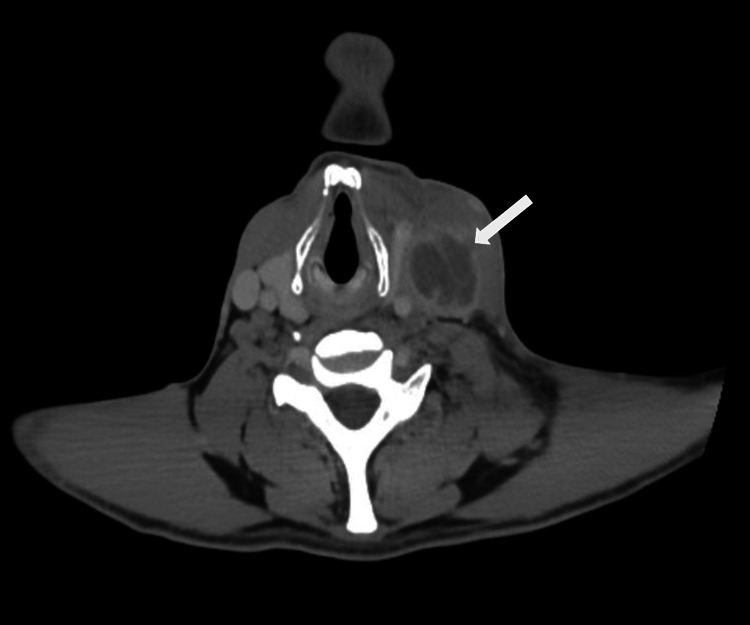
Contrast-enhanced CT of the neck from a previous admission showing left internal jugular vein thrombosis (white arrow) at the level of the upper cervical region related to initial episode of Lemierre's syndrome
